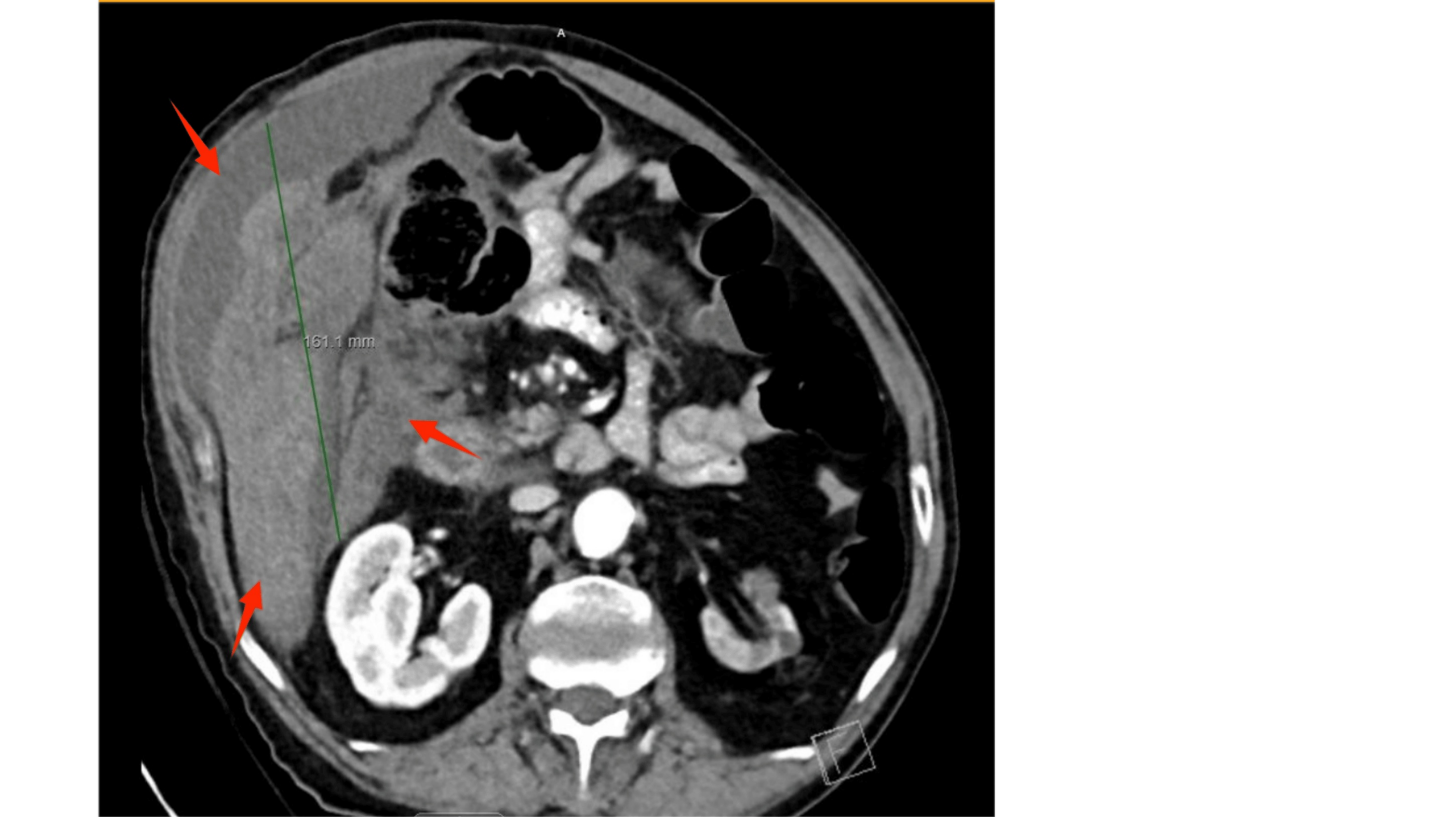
Axial abdominal CT scan showing large right intraperitoneal hematoma Contrast-enhanced axial CT image demonstrates a large right-sided intraperitoneal hematoma measuring approximately 161 mm (16.1 cm) in craniocaudal length. The arrows indicate hematoma.
